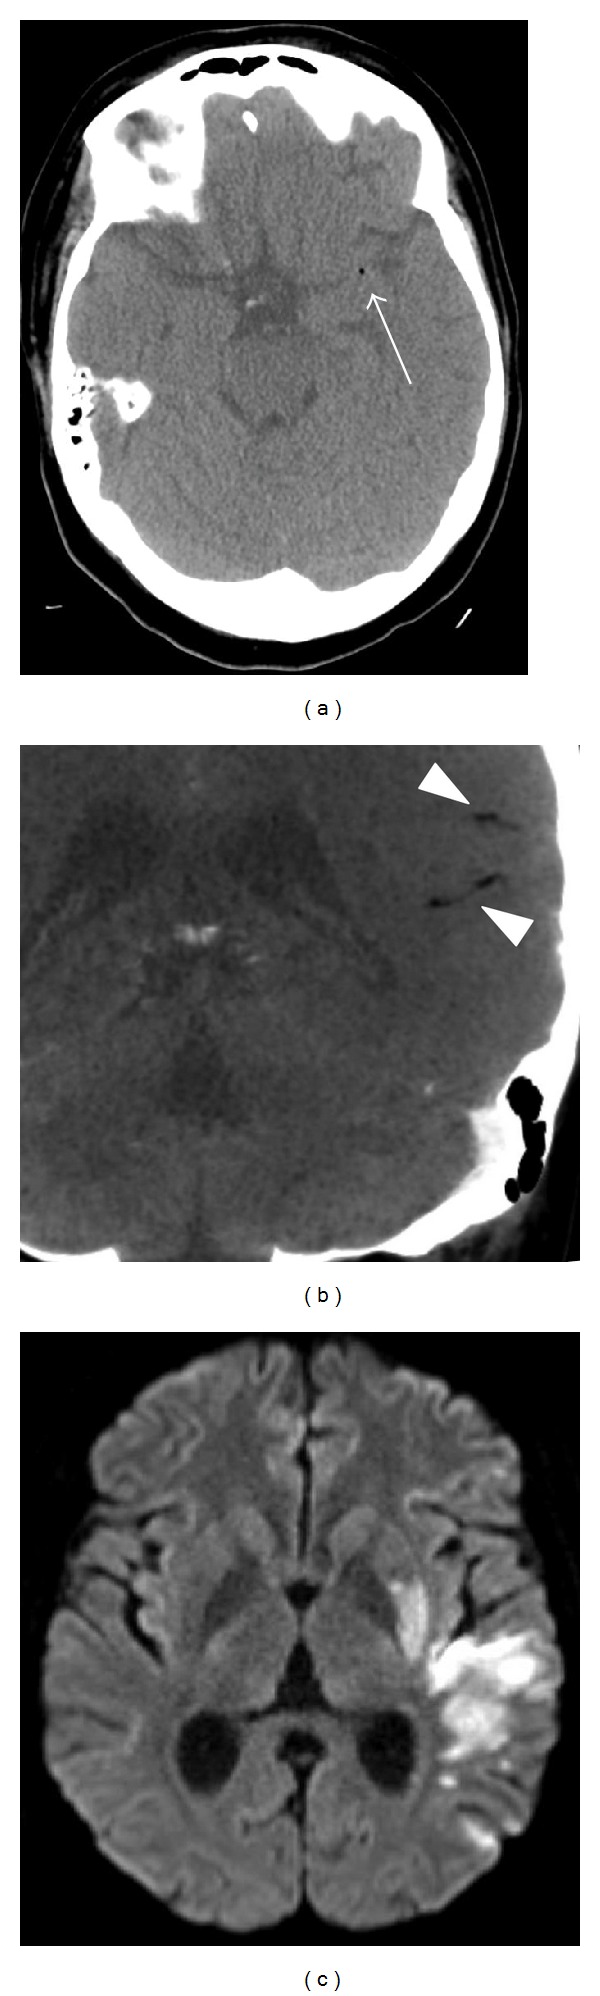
Air emboli on CT (a), CTA (b), and infarction on DWI sequence of MRI (c).
